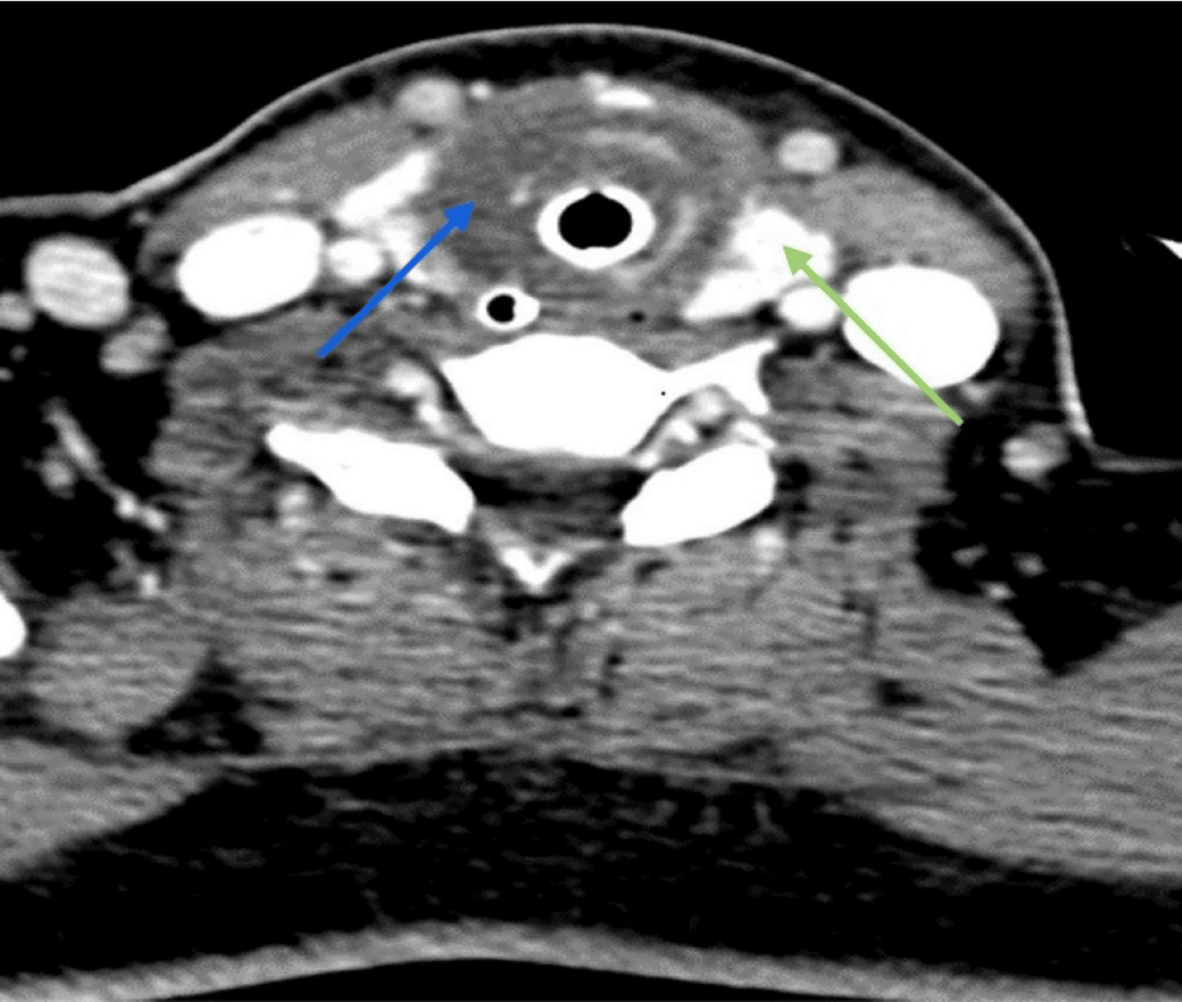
CT axial view shows circumferential thin fluid seen surrounding the trachea (blue arrow), adjacent to the thyroid gland (green arrow).
